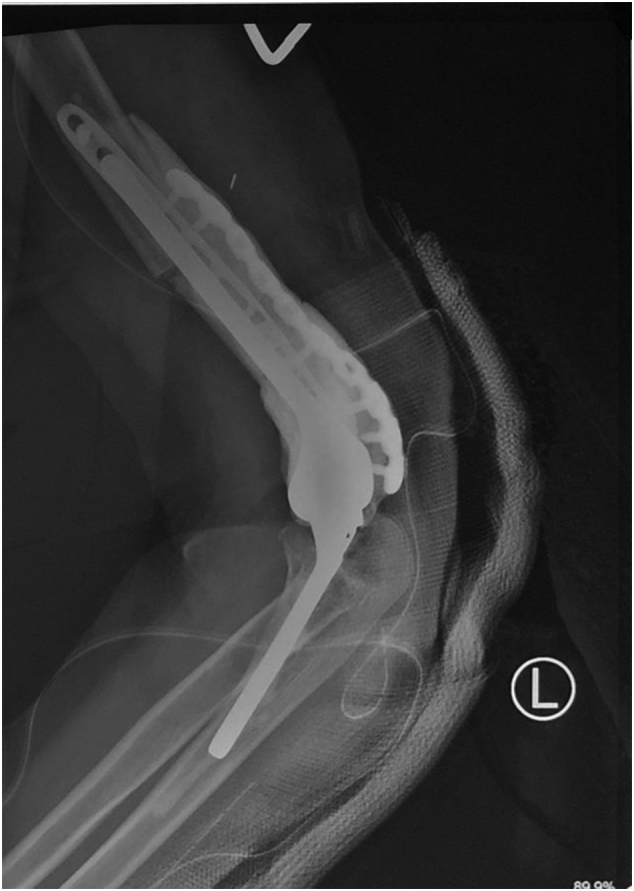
Postoperative X-ray of left humerus.
